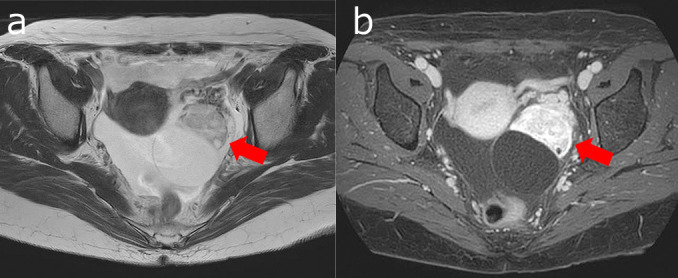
Magnetic Resonance Imaging of the pelvic region (Case 1) A 96-mm sized solid tumor with some cystic components was found in the left pelvis. T2-weighted imaging revealed isointense and hyperintense signal areas with strong contrast enhancement. Although ovarian malignancy was suspected, it was difficult to differentiate primary and metastatic ovarian tumor. a: T2-weighted horizontal section, b: T1 contrast-enhanced horizontal section
